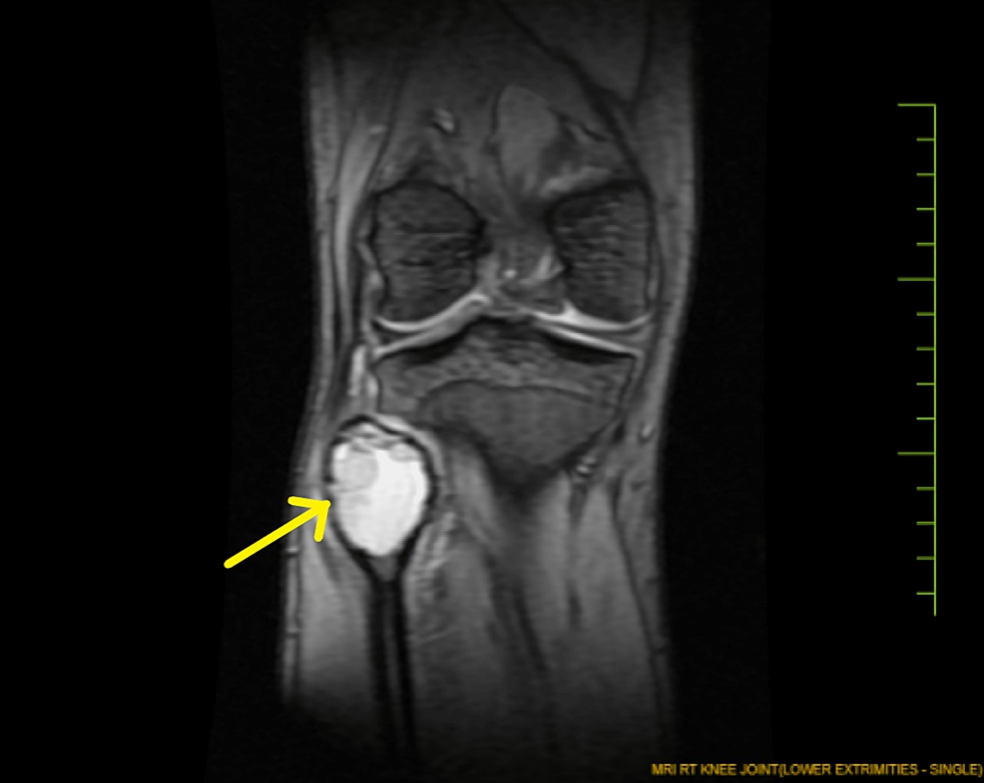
Magnetic resonance imaging of the right knee. The arrow marks the expansile cystic lesion involving the right fibular head. It shows the thinning of the cortex.
